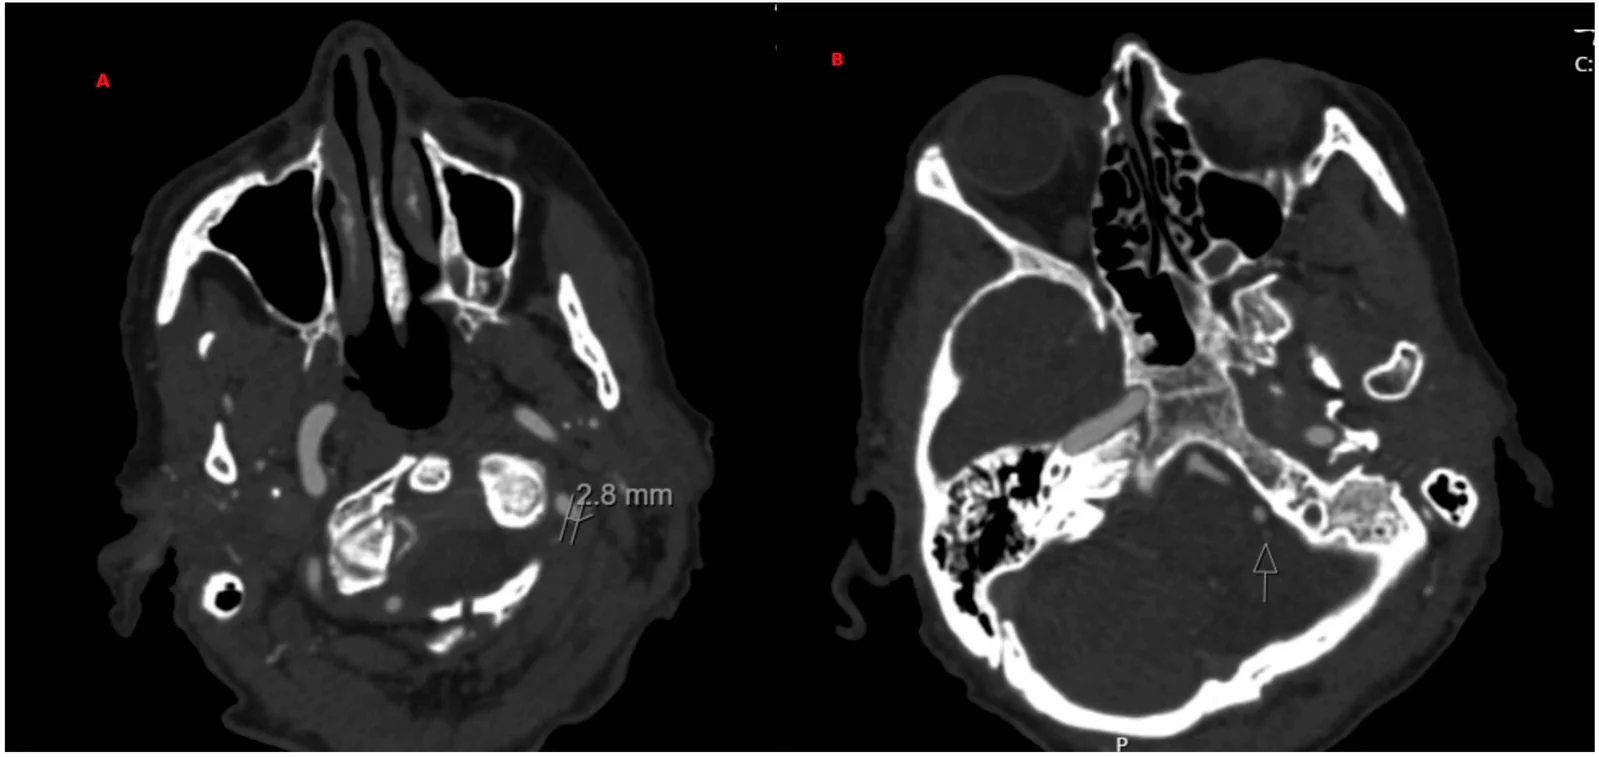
Correlative CT findings. (A) Axial CTA source image at C1-C2 shows chronic atlantoaxial deformity, peri-odontoid soft tissue, and a C1 arch abnormality. The 2.8 mm caliper annotation is a source-image measurement in the left V3 region. (B) Axial noncontrast head CT shows left cerebellar hypoattenuation (arrow), corresponding to the dominant infarct. P denotes posterior. Peripheral text and annotations are retained from the source images.
